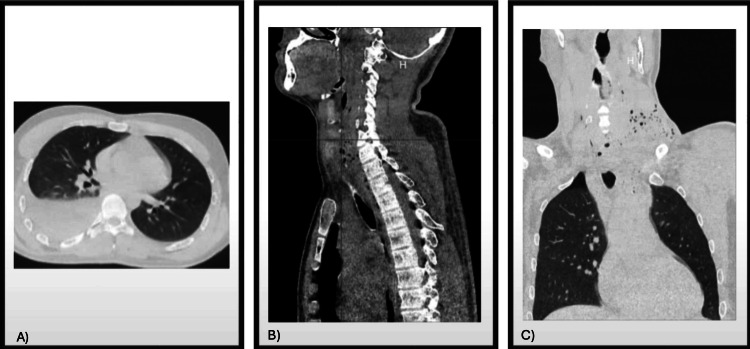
(A) Axial section of a simple and contrast-enhanced chest CT scan showing the presence of a right-sided hemopneumothorax, with evident air-fluid level and associated pulmonary contusion. (B) Sagittal view of a simple and contrast-enhanced CT scan of the neck and thorax. (C) Coronal view of a simple and contrast-enhanced CT scan of the neck and thorax. In both sections (B and C), subcutaneous emphysema is observed along the bullet trajectory through the cervical region, without evidence of vascular or spinal lesions. Additionally, image 1C also shows the right-sided hemopneumothorax.
